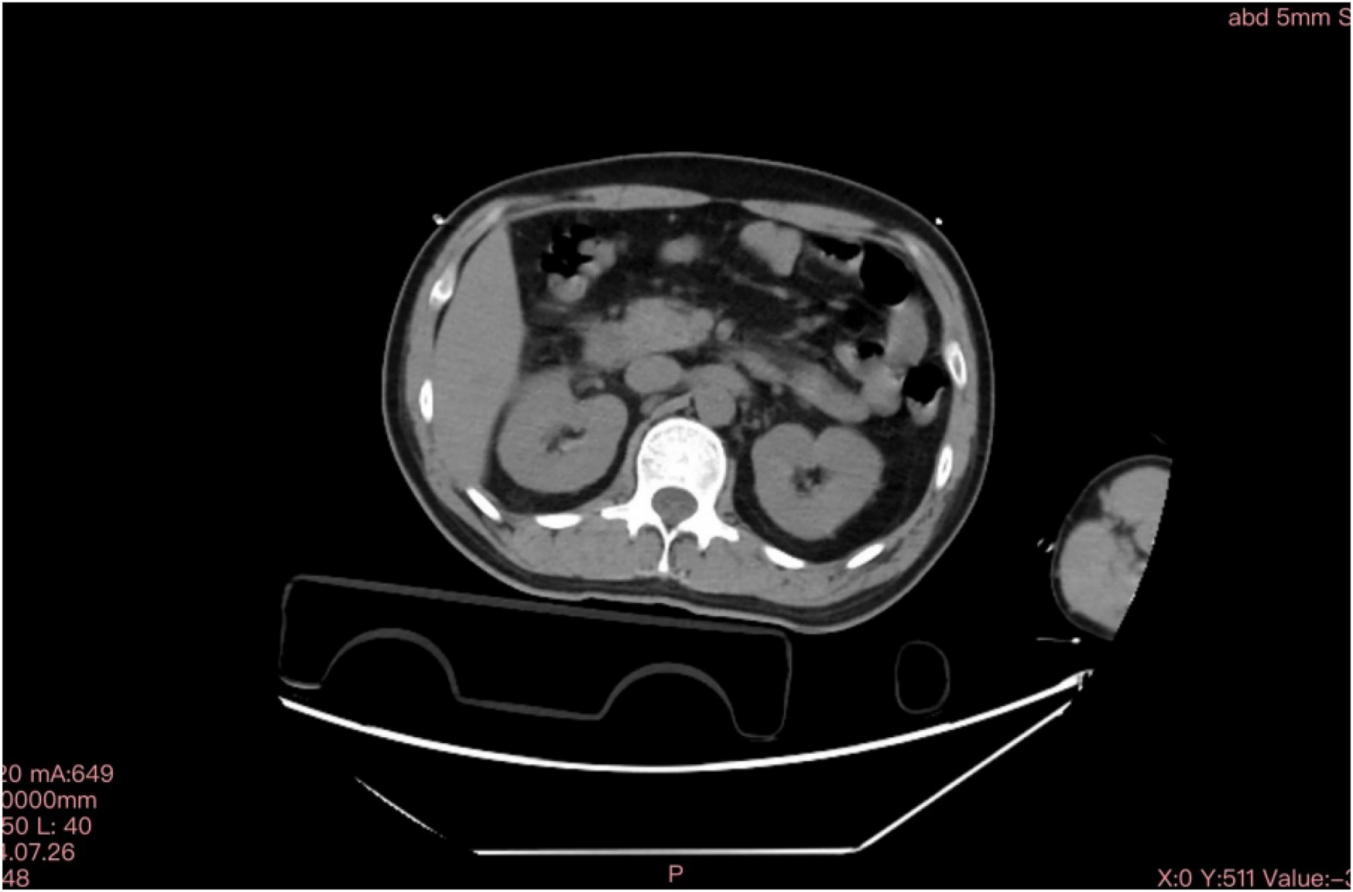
Abdominal computed tomography scan on July 26,2024. Swollen pancreas without gallbladder stones.
